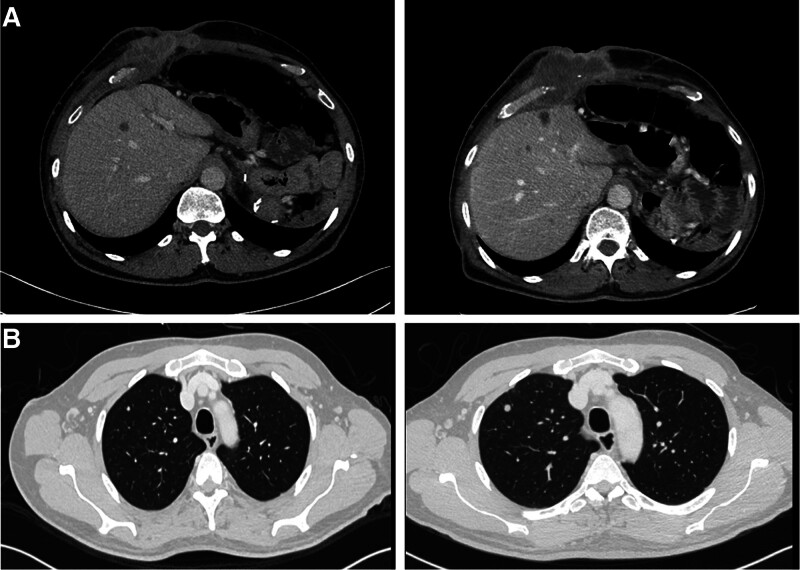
(A.1) Axial CT scan shows interval recurrence at surgical bed with new liver lesion. (A.2) Axial CT scan shows interval progression at surgical bed with new liver lesions. (B.1) Axial CT scan lung window setting shows a new lung nodule seen in the right upper lobe. (4B.2) Axial CT scan lung window setting shows interval increase in size of the right upper lung nodule. CT = computed tomography.
